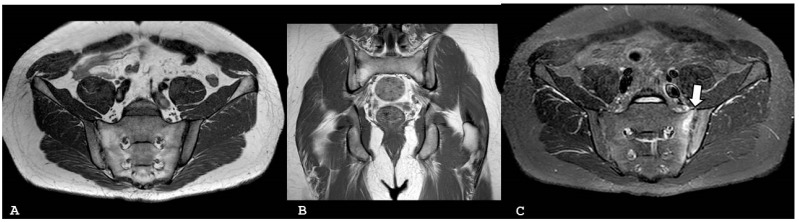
Oblique coronal (A) and coronal (B) T1-weighted magnetic resonance imaging sequences show that the excavated iliac bone of the right sacroiliac joint is replaced by tissue showing MR signal intensity similar or higher than normal bone marrow (backfill). New fat metaplasia in the subchondral bone marrow of the right sacral and iliac bones is also evident. Oblique coronal short tau inversion recovery (C) sequence demonstrates abnormal signal intensity in marrow adjacent to the left sacroiliac joint suggestive of sacroiliitis. Capsulitis can be visible anteriorly (arrow). Note synovitis with increased signal intensity in the joint space of the left sacroiliac joint. The non-active involvement of the right sacroiliac joint.
